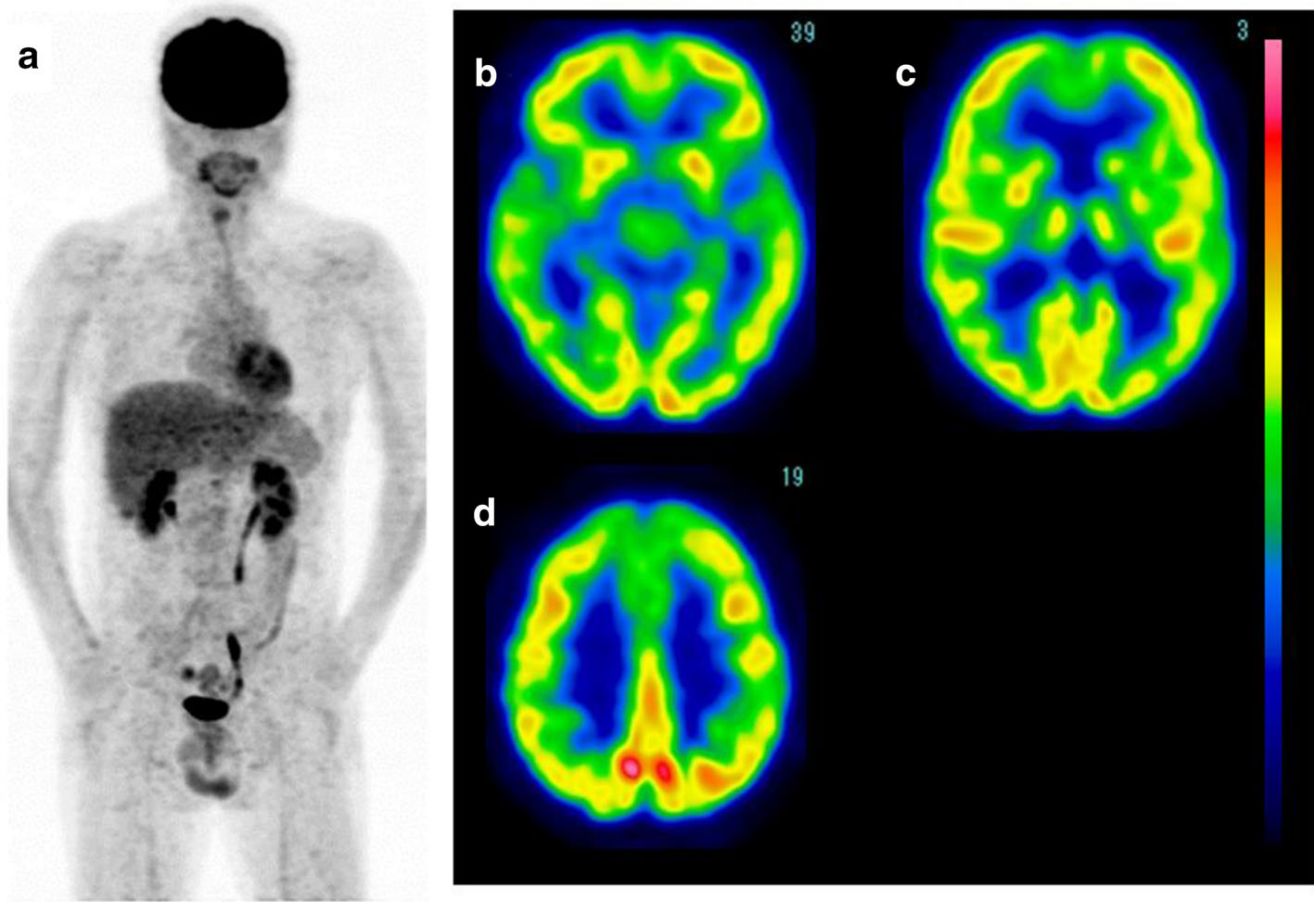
After chemotherapy, the abnormal FDG uptake in these areas completely disappeared (a) and the cerebral glucose metabolism improved (b–d)
